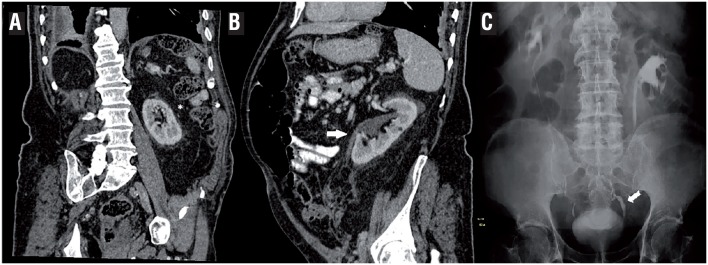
A) Coronal-oblique post-contrast-enhanced abdominal CT scan showing left pelvic kidney (asterisk) without dilated ureter after correction of the inguinal hernia. B) Sagittal CT scan showing non dilated left pelvic kidney (arrow). C) Intravenous pyelogram post hernioplasty.
